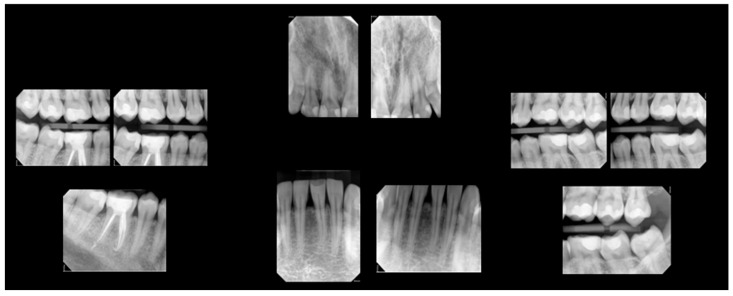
Two-year follow-up radiographs.
